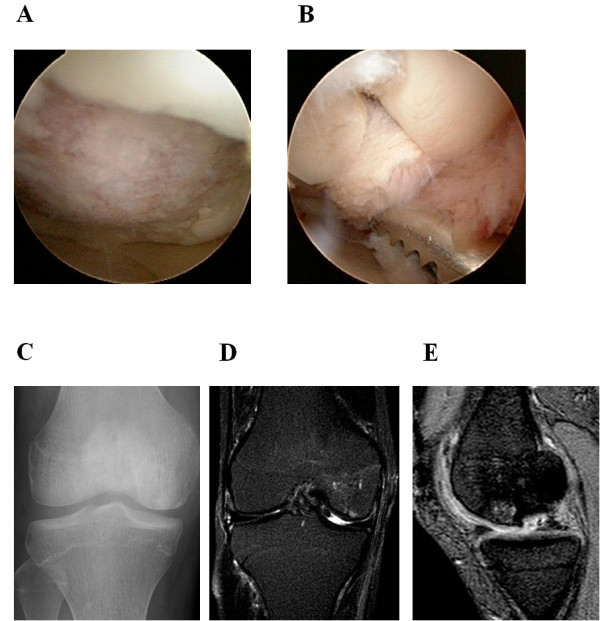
Operative and postoperative findings in case 3. (A) Arthroscopy showing the lesion of the osteonecrosis at the medial condyle of the femur demonstrating the anterior wide margin of the femoral condylar defect. (B) Osteochondral plugs are grafted into the recipient site. (C) Anteroposterior radiograph taken six months after surgery shows less irregularity of the subchondral bone of the medial femoral condyle. (D, E) MRI taken six months after surgery shows the restoration of the articular cartilage surface and good engraftment of the graft.
